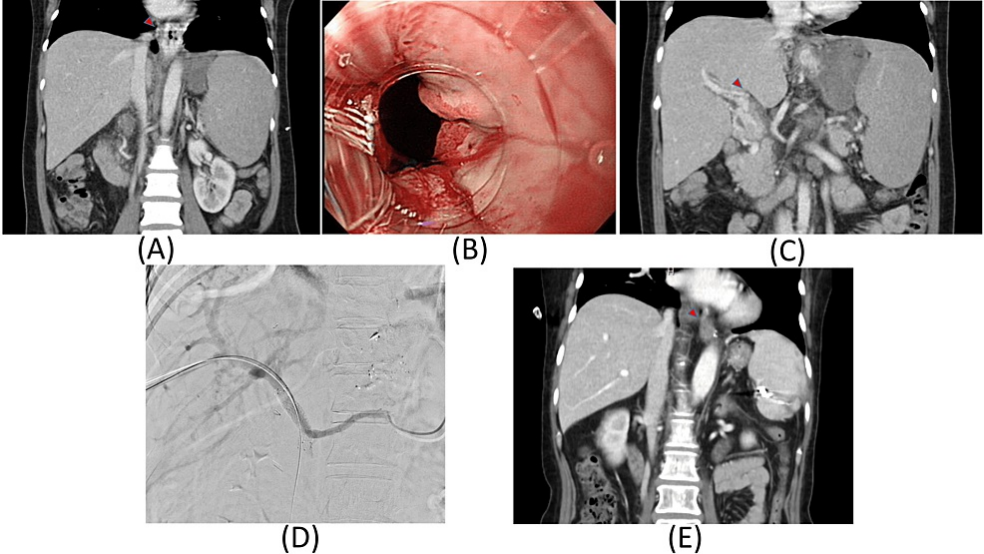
CT scans and intraoperative view of the portal vein stent insertion procedure A: Coronal view of the CT scan showing splenomegaly and varices but no sign of cirrhosis; B: Esophagogastroduodenoscopy showing esophageal varix with 'white nipple sign' at the gastroesophageal junction; C: Coronal view of the CT scan showing portal vein thrombosis; D: Portal vein stent insertion procedure; E: Coronal view of the CT scan showing resolution of previously noted varices
